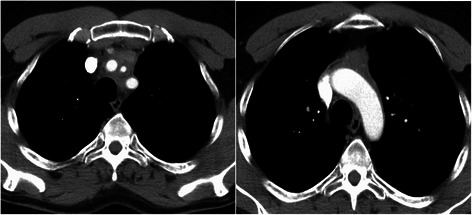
CT-scan of patient no. 5 with thymic carcinoma (WHO C) encircling the supraaortic branches and with suspicious invasion of the aortic arch
